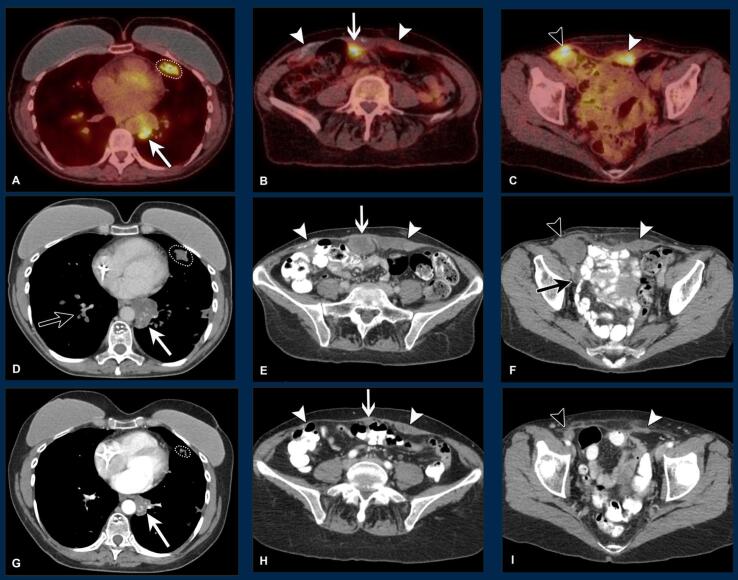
Radiology images showing response over time in the chest, abdomen, and pelvis A-C: Pretreatment 18F-FDG PET/CT imaging at initial workup: Metastatic disease seen as avid pulmonary nodules (A, circle); partially necrotic infrahilar mass (A, white closed arrow); peritoneal mass along abdominal wall (B, white open arrow); external iliac node (C, black arrowhead); and patchy areas of avidity within confluent thickening of the rectus abdominis muscles, where there are also dystrophic calcifications (B and C, white arrowheads). D-F: Axial post-contrast CT imaging eight months later: Progression after EMA/EP seen as continued pulmonary nodules (D, circle); enlarging infrahilar mass (D, white closed arrow; 4 cm, previously 3.4 cm); peritoneal mass (E, white open arrow; 4.1 cm, previously 2.8 cm); external iliac node (F, black arrowhead; measuring 3.9 cm, previously 2.7 cm); and continued rectus abdominis thickening (E and F, white arrowheads). Note also tumoral and bland pulmonary emboli (D, black closed arrow) and deep venous thrombosis (F, black closed arrow) from invasion of the right external iliac vein at the level of the external iliac node. G-I: Axial post-contrast CT imaging an additional five months later: Response with pembrolizumab with decreased pulmonary nodules (G, circle); infrahilar mass (G, white closed arrow; 2.3 cm, previously 4 cm); peritoneal mass (H, white open arrow; 1 cm, previously 3.9 cm); right inguinal node (I, black arrowhead; measuring 1.5 cm, previously 3.9 cm); and rectus abdominis thickening (H and I, white arrowheads).
